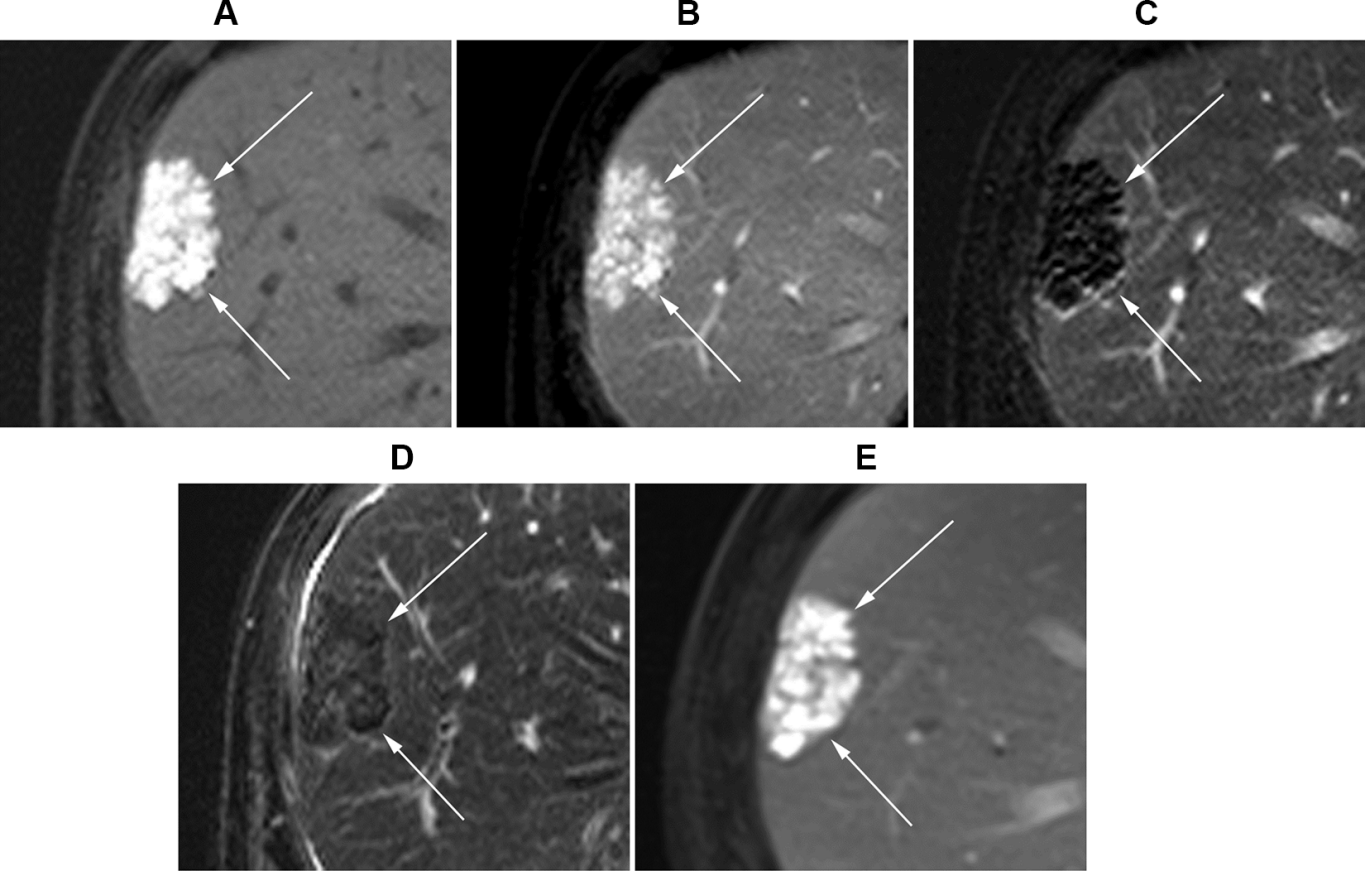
Gadoxeticacid-enhanced liver MR images of a 56-year-old male with uveal melanoma (A) Precontrast T1-weighted image. (B) Post-contrast portal venous phase T1-weighted image. (C) Subtraction image (portal venous phase – precontrast T1-weighted image). (D) T2-weighted image. A 4.6 cm metastatic melanoma lesion (arrows) was observed in segment 8, which was graded to show negative enhancement and low T2 SI. (E) In follow-up MRI T1-weighted post-contrast-enhanced portal venous phase image, the lesion measured 4.3 cm.
